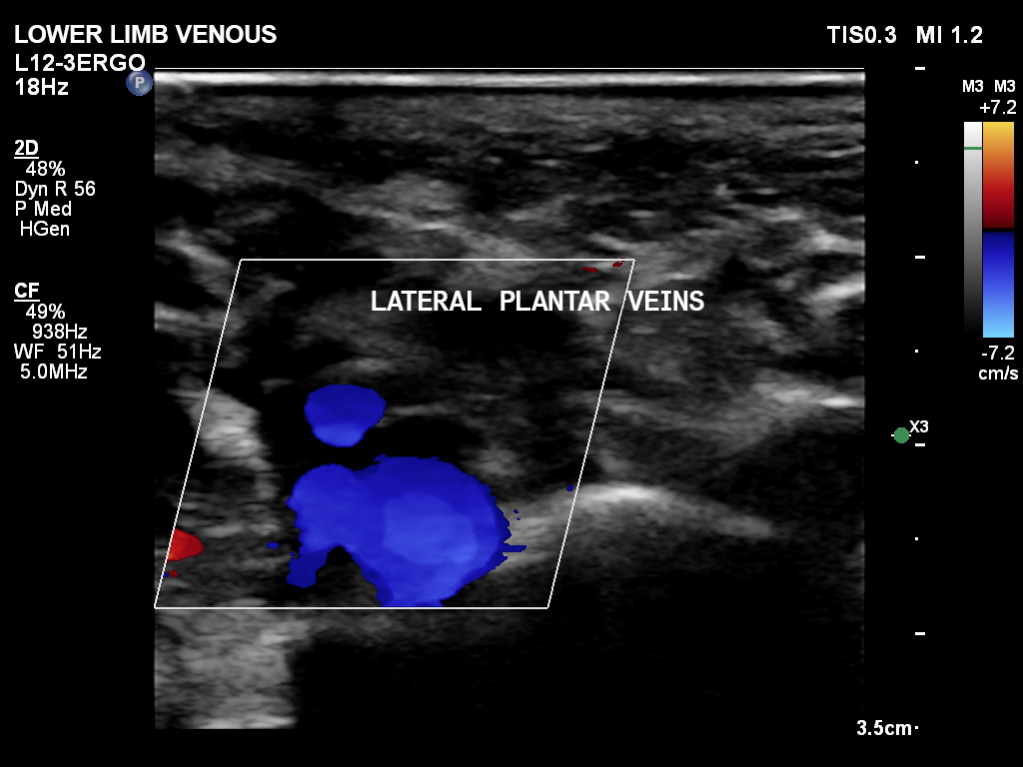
Control plantar vascular ultrasound of the right lower limb at 3 months, in transverse view, demonstrating complete recanalization of the prior thrombus.
